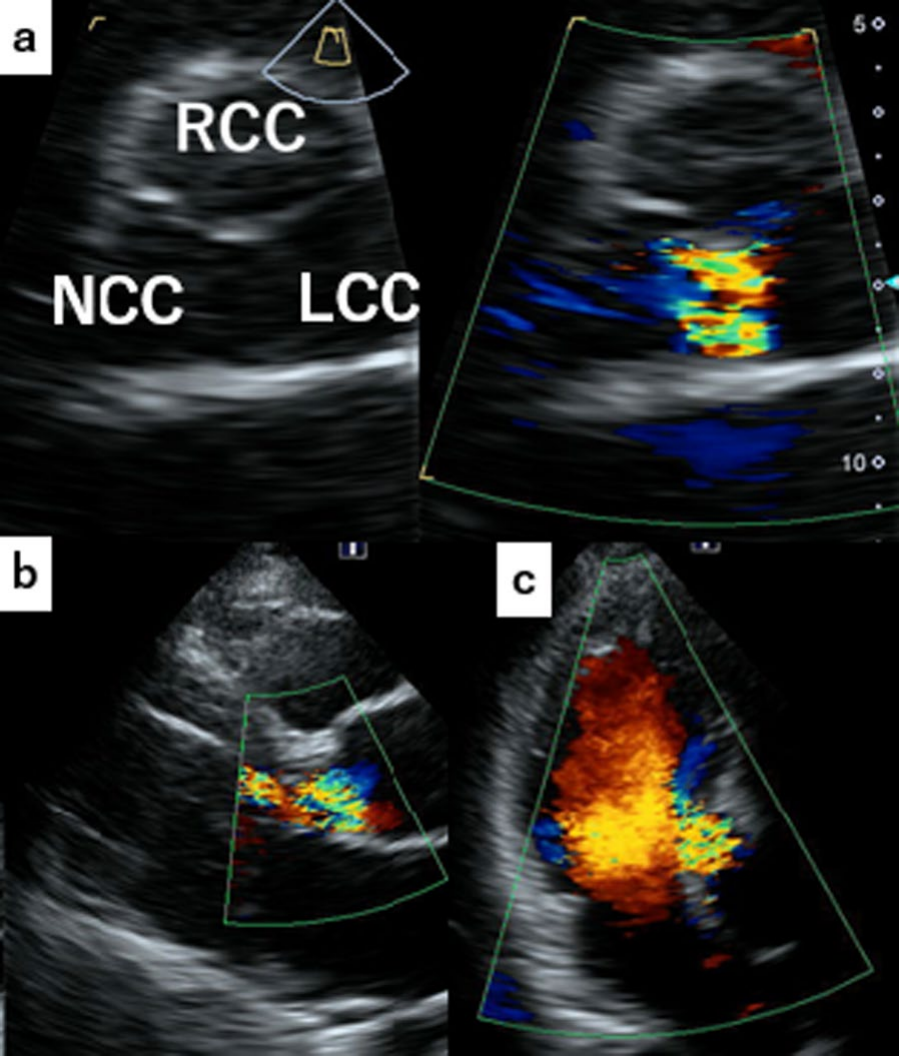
Echocardiographic findings of aortic valve and regurgitation. Transthoracic echocardiography in parasternal short-axis (a), long-axis (b), and three-chamber (c) views. The jet of severe aortic regurgitation is directed toward the left ventricle between the noncoronary cusp (NCC) and the left coronary cusp (LCC). The right coronary cusp (RCC) is also indicated
